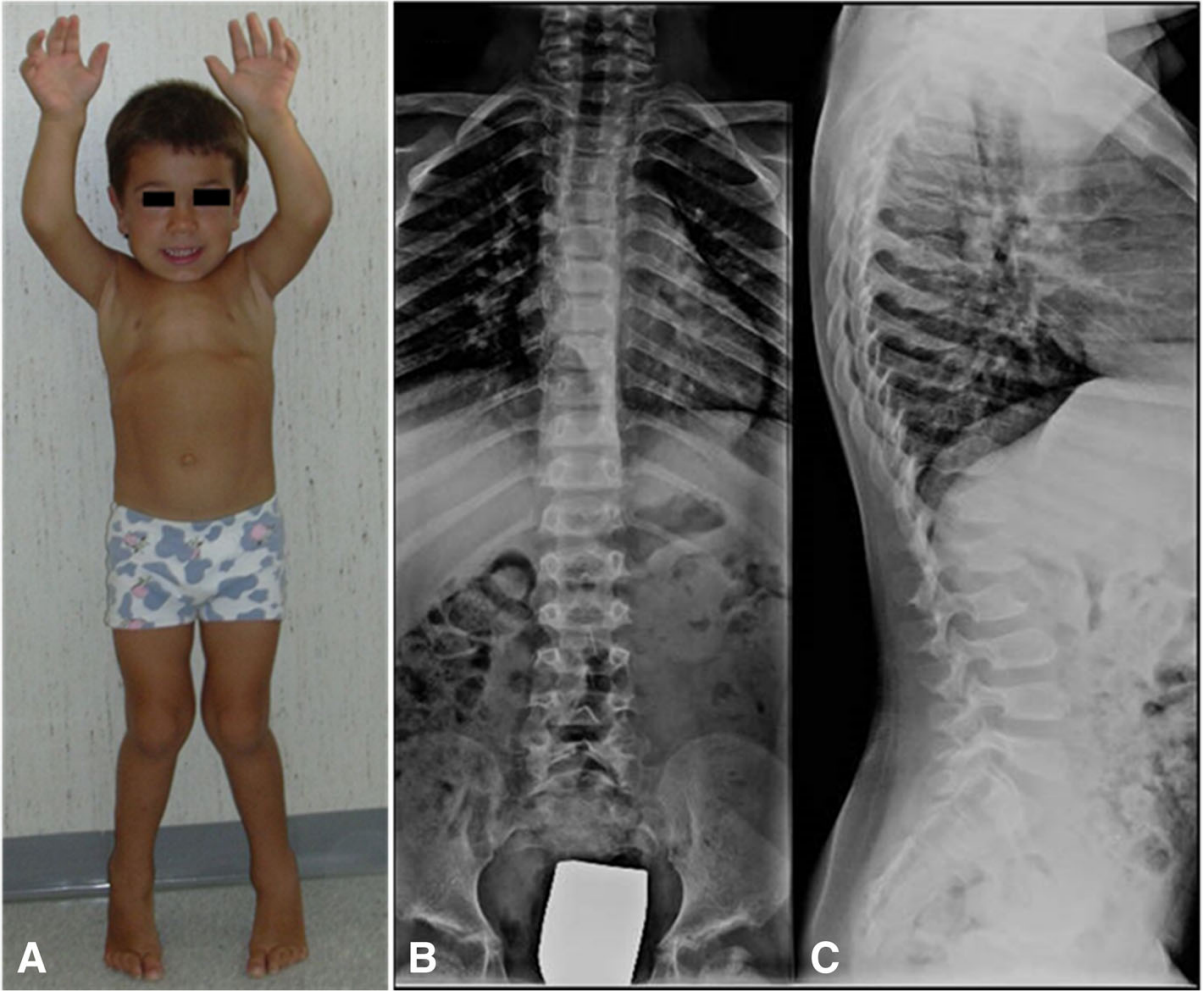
MPS IVA. a 4 year-old patient with b antero-posterior and c lateral x-rays showing “paddle-shaped” ribs and flattened and rounded vertebrae with a typical “anterior beaking” aspect
